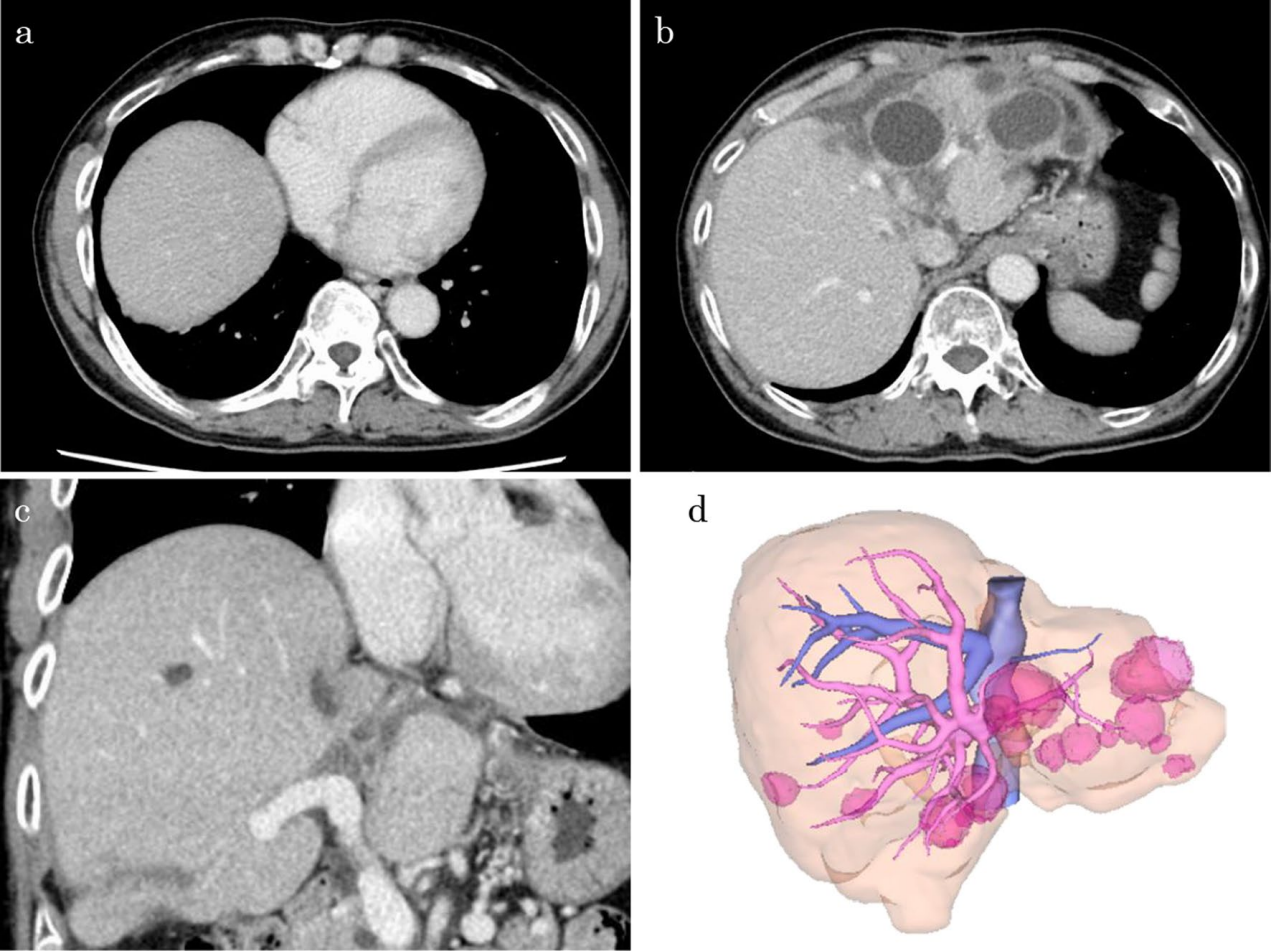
Computed tomography performed 6 months after the surgery reveals no recurrence of the right heart decompression (a), inferior vena cava stenosis (b), or portal vein stenosis (c). The 3D reconstructed image shows the disappearance of the cyst near the IVC and RHV (d)
